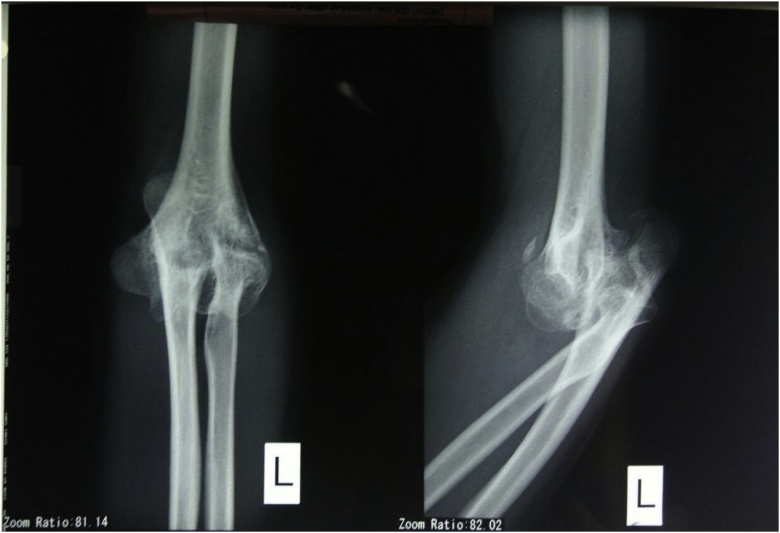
X-ray of the left elbow AP and lateral.
